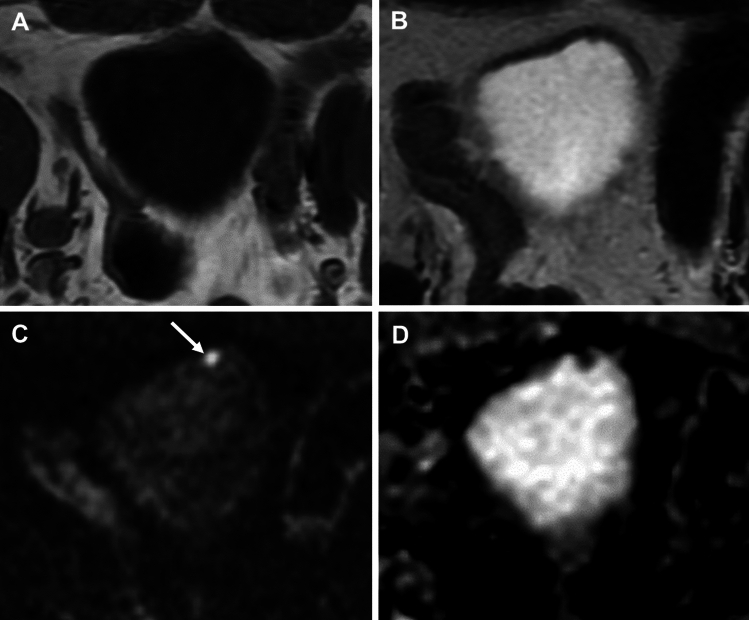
Axial T1WI (A), T2WI (B), DWI (C) and ADC map (D) in a 68-year-old male with urothelial carcinoma who underwent MRI after TURBT shows a 5 mm lesion at the anterior bladder wall to the left of the midline close to site of known multifocal tumour seen at TURBT (pT1G3). This lesion was missed on the anatomical sequences (A, B) but the focal hyperintense area at DWI (arrow) and corresponding restricted diffusion on ADC maps (C, D) allowed this to be called with the combined read. Cystectomy confirmed tumour in this location, pTis disease
